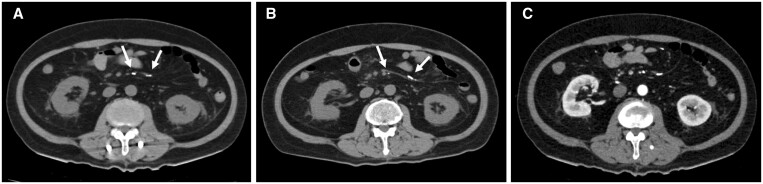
Axial CT images captured after transcatheter arterial embolization (TAE). (A) Plain CT image captured immediately after the first TAE. (B) Plain CT image captured 1 month later. These images depict the migration of *N*-butyl-2-cyanoacrylate glue to the distal ileal artery 1 month post-TAE (arrows). (C) Contrast-enhanced CT (arterial phase) image at the same level as the plain CT image shown in (A). The *N*-butyl-2-cyanoacrylate glue was masked by the contrast medium, making it difficult to distinguish from normal vessels.
